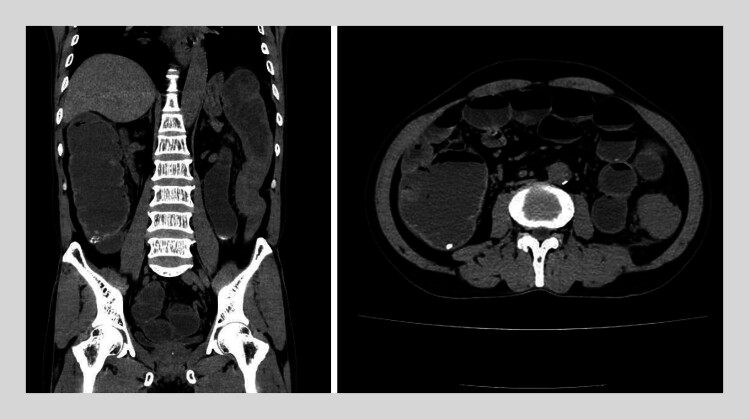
Computed tomography (CT) image of descending colon cancer. The CT image demonstrated wall thickening of the descending colon and dilation of the proximal colon and small intestine.
